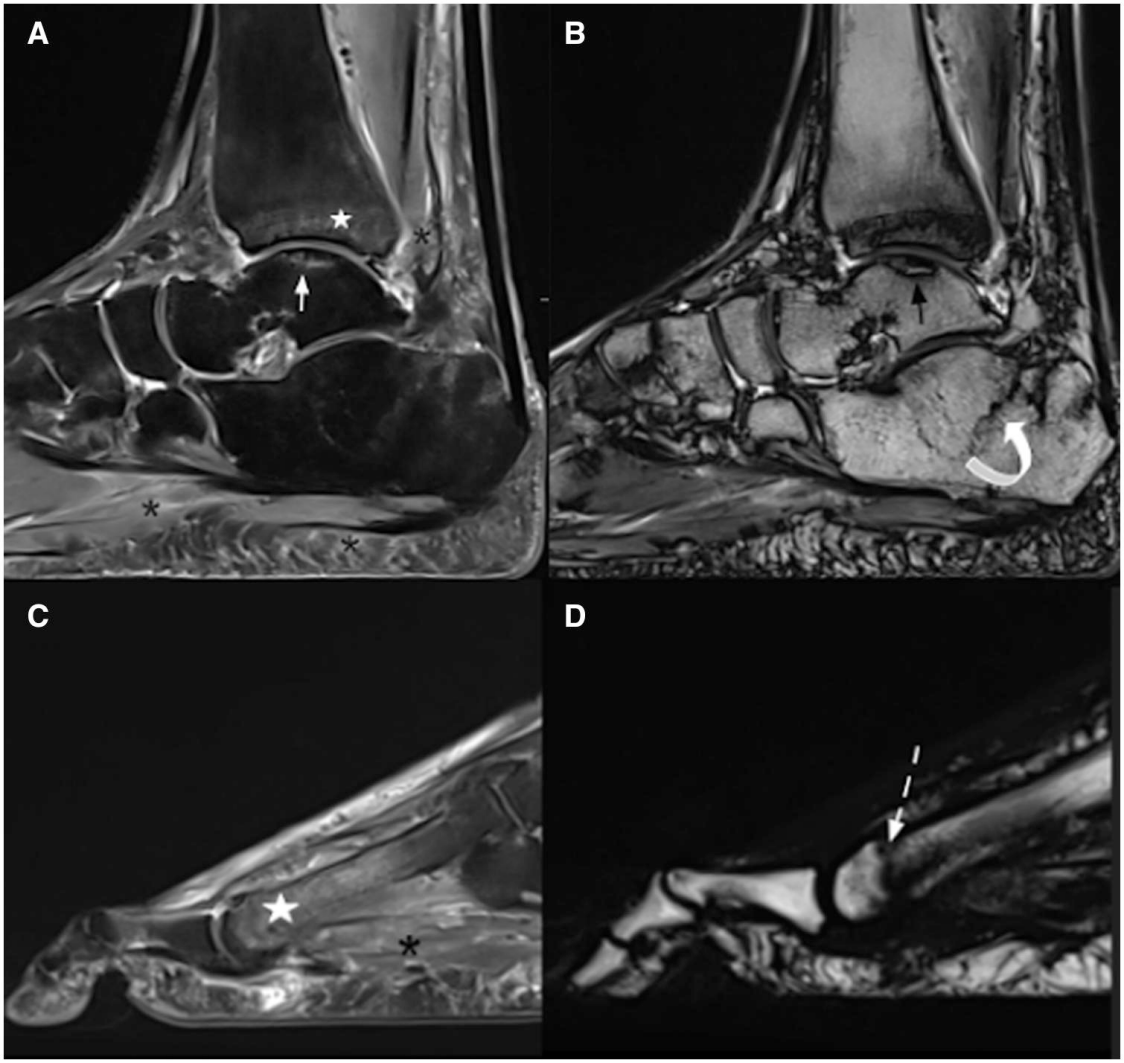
MRI images of the left ankle and foot shows talar dome and metatarsal distal shaft fractures soft tissue and bone marrow oedema—fracture of the talar dome (white arrow), marrow oedema in distal tibia (star), and soft tissue and kager’s fat pad oedema (asterisk) on sagittal PDFS image (A), subchondral insufficiency fracture of the talar dome with surrounding sclerosis (black arrow) and hypointense fracture line in calcaneus (curved arrow) on sagittal T2W image (B), oedematous changes in the shaft of metatarsal (star) and oedematous changes in surrounding soft tissue (asterisk) on sagittal PDFS image (C), hypointense fracture line in distal shaft of metatarsal (dashed arrow) on sagittal Dixon fat only image (D).
